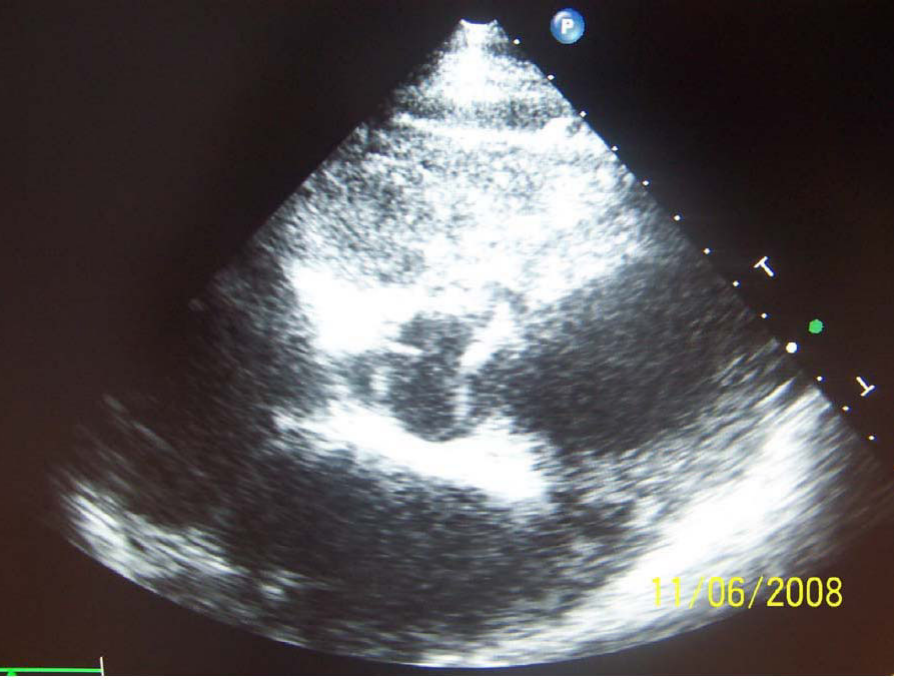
Short axis parasternal view, bicuspid aortic valve.
